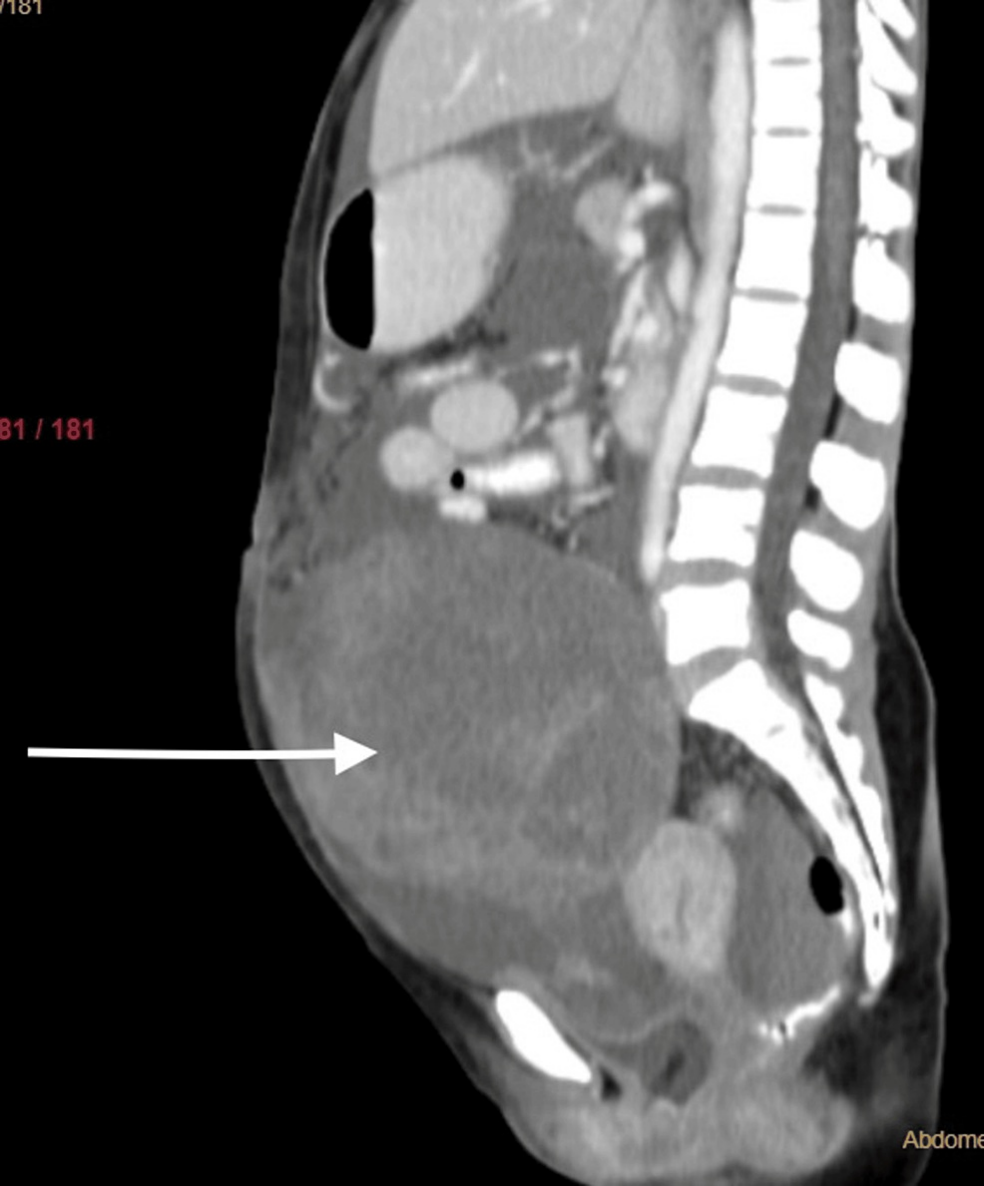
Contrast-enhanced CT abdomen and pelvis sagittal section solid-cystic mass in the right adnexal region (white arrow)
